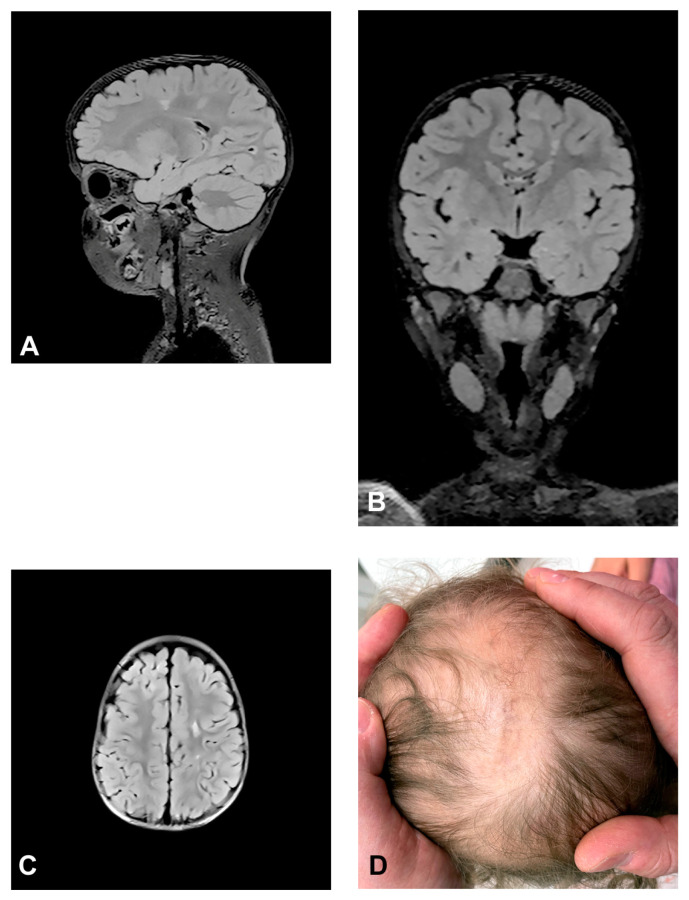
(Case 3) Sagittal (A), coronal (B) and axial (C) T2 FLAIR magnetic resonance images at the age of 2.5 showing small foci of hyperintensities, which appear in a band and triangle-like shape, spreading from subcortical white matter to the left lateral ventricle similarly to radial migration lines. (D) IP skin changes in the form of Blaschko lines on the same side of the scalp where the small foci of hyperintensities were found.
